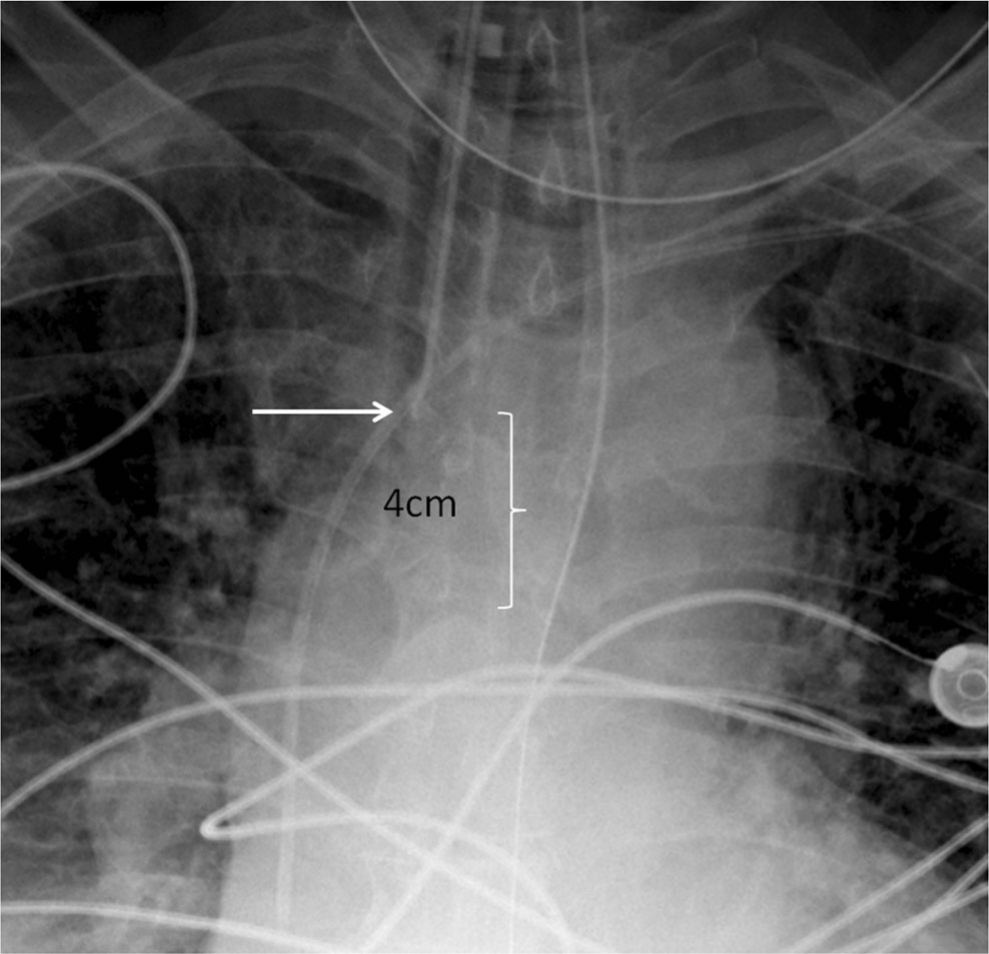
Cropped chest radiograph image shows that the ET tube tip (*white arrow*) is in satisfactory position 4 cm above the carina
